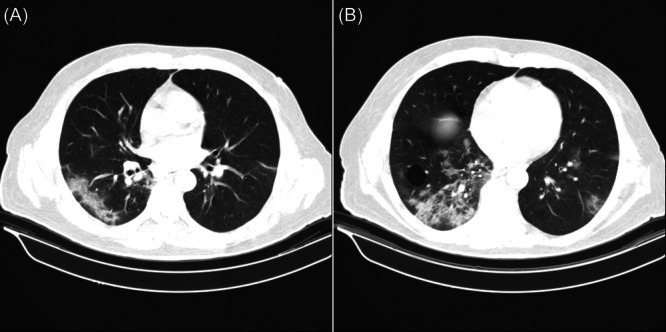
Chest CT scan of an immunocompetent patient with ground glass and consolidation pattern of lesions. These Pictures, depict a 54‐year‐old immunocompetent male patient with involvement of Right lower and left lower lobes, with central, peripheral, and anteroposterior locations of lesions, and both wedged and confluent shapes of lesions and mixed patterns of ground glass and consolidation which were significantly higher among immunocompetent patients. The right lower lobe scored 4/5 and the left lower lobe scored 3/5 (Shown in A and B) based on the semiquantitative scoring system and with 1/5 for the other three lobes with the sum of 10/25.
